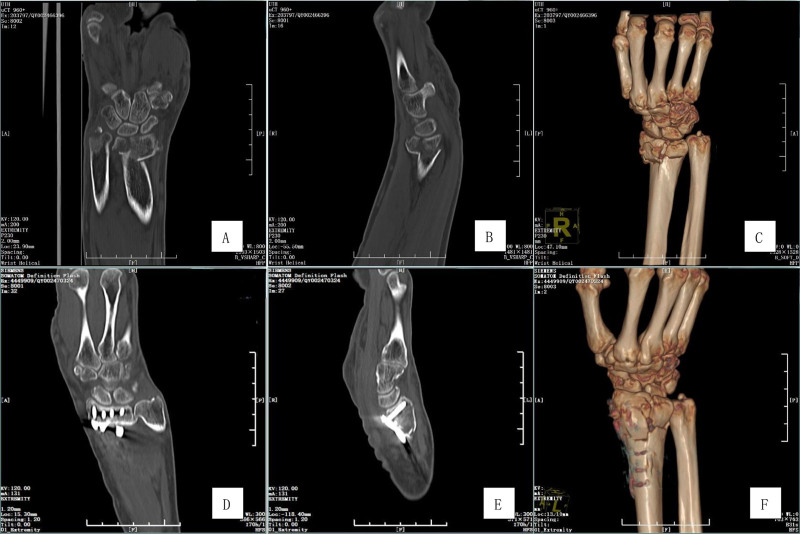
Imaging findings before surgery and 3 days after surgery. (A) Preoperative coronal CT revealed multiple fracture lines on the articular surface of the distal radius. (B) Preoperative sagittal CT indicated that the fracture of the distal radius articular surface is separated and exhibits steps. (C) Preoperative 3-dimensional CT demonstrated a fracture of the distal radius with dorsal comminution. (D) The coronal CT scan 3 days postoperation showed that the articular surface of the distal radius is flat, and the screws were evenly distributed. (E) The sagittal CT 3 days postoperation revealed that the articular surface of the distal radius had been reduced. The screws were positioned close to the articular surface, providing support, and their length extended just below the dorsal bone cortex. (F) The 3-dimensional CT 3 days postoperation indicated that the comminuted fracture on the dorsal side had been largely reduced. CT = computed tomography.
